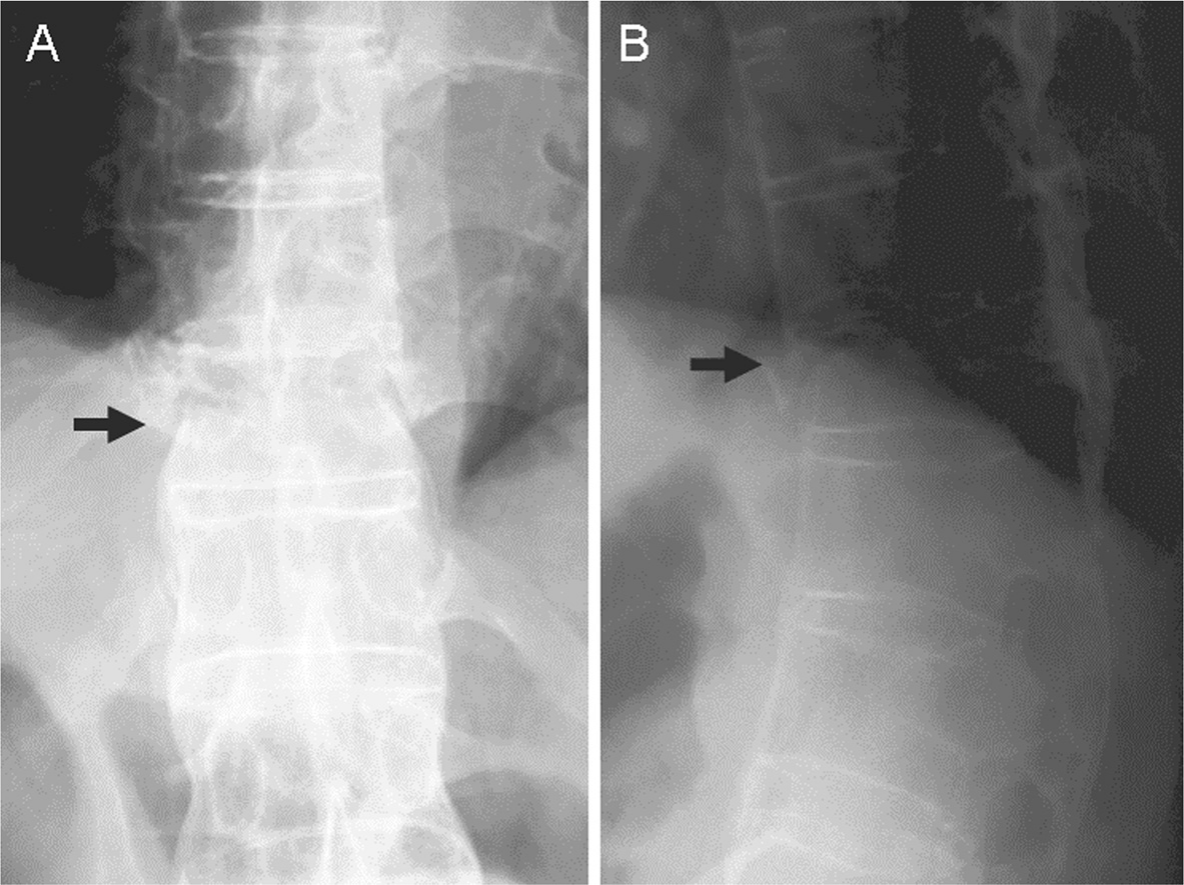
Anteroposterior (A) and lateral (B) radiographs taken when a patient with Parkinson’s disease was first referred to a local hospital four days after onset of back pain. Fracture of the T11 vertebral body (arrow) was suspected.
